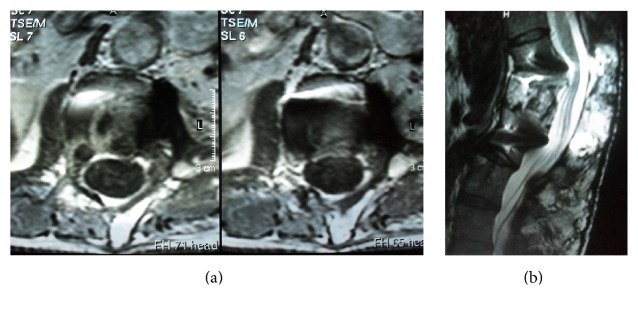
At the fifth year follow-up MRI, axial T_1_WI with gadolinium and sagittal T_2_WI show no recurrence.
